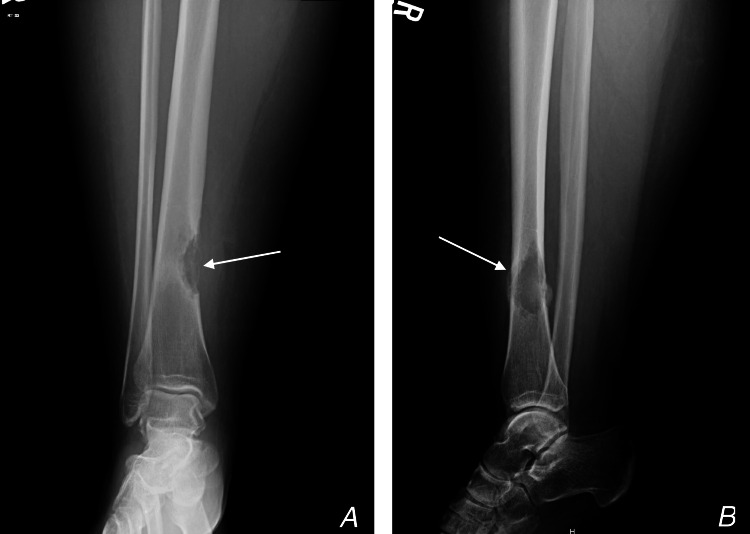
X-ray images of the patient A: Shows an anterior-posterior view of a right distal tibia, B: Shows a lateral view of the distal tibia. Arrows showing a well-circumscribed, cortical tumor with a multilobulated, osteolytic pattern.
